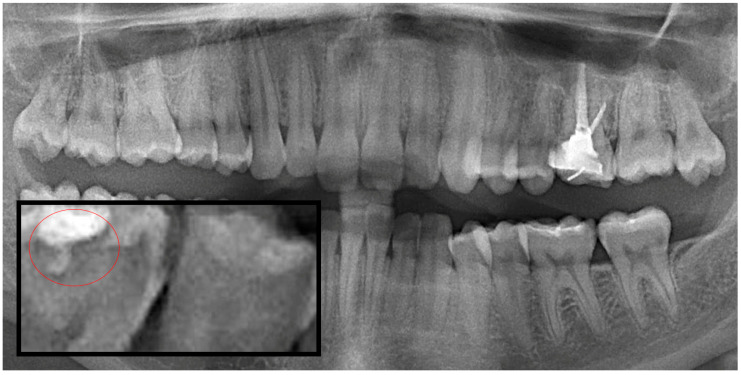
Panoramic radiography and segmented secondary caries, red circle show the caries.
